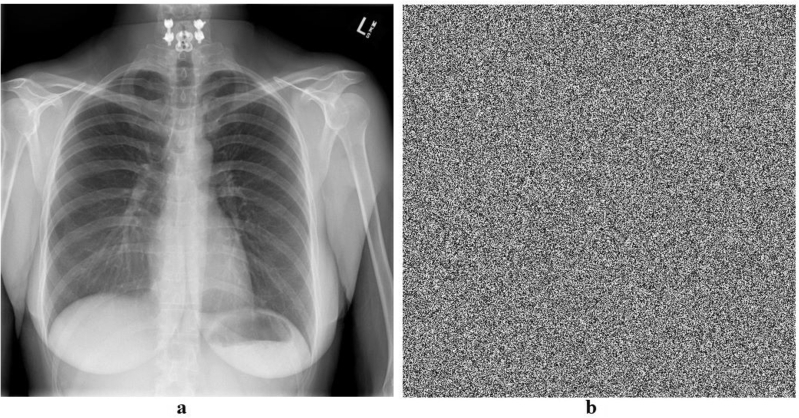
Plain and encrypted Xray image.
